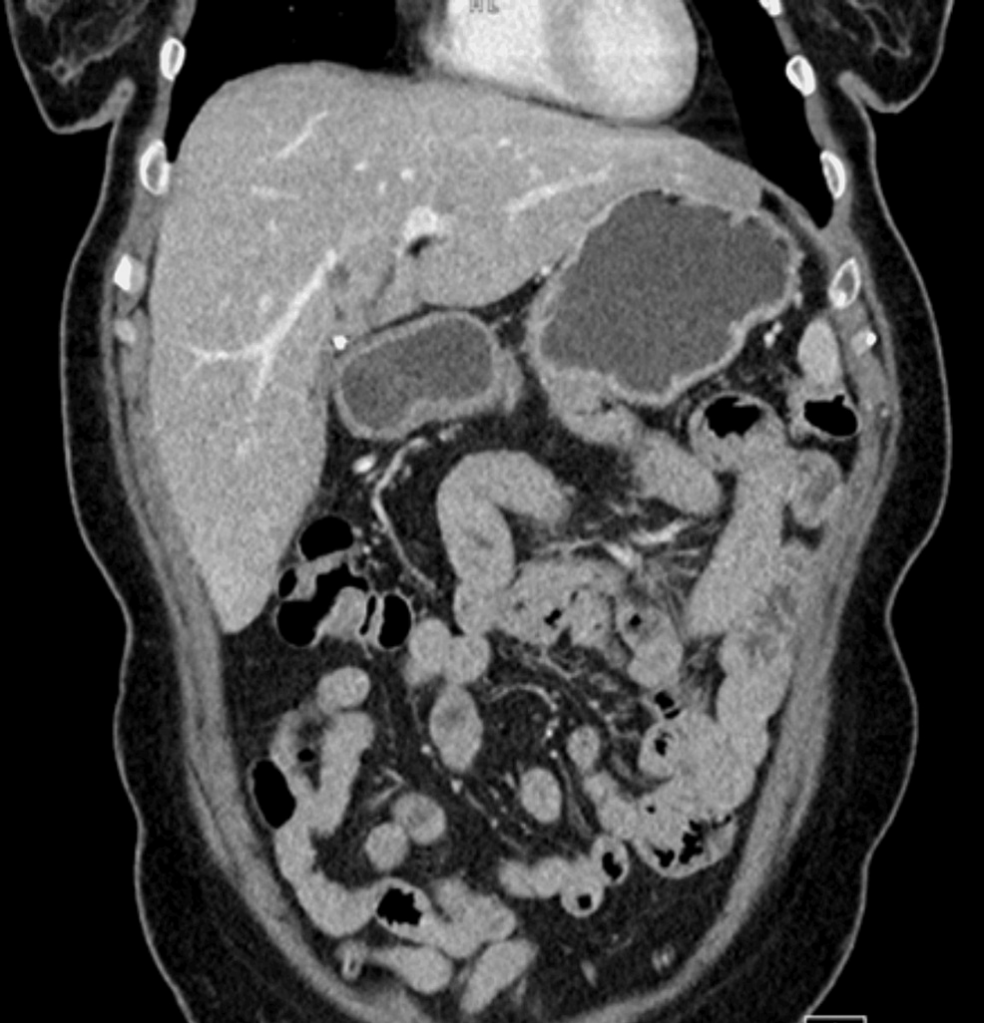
CT of the abdomen and pelvis four months postoperatively showing resolution of the multicystic mass and no evidence of lymphadenopathy.
